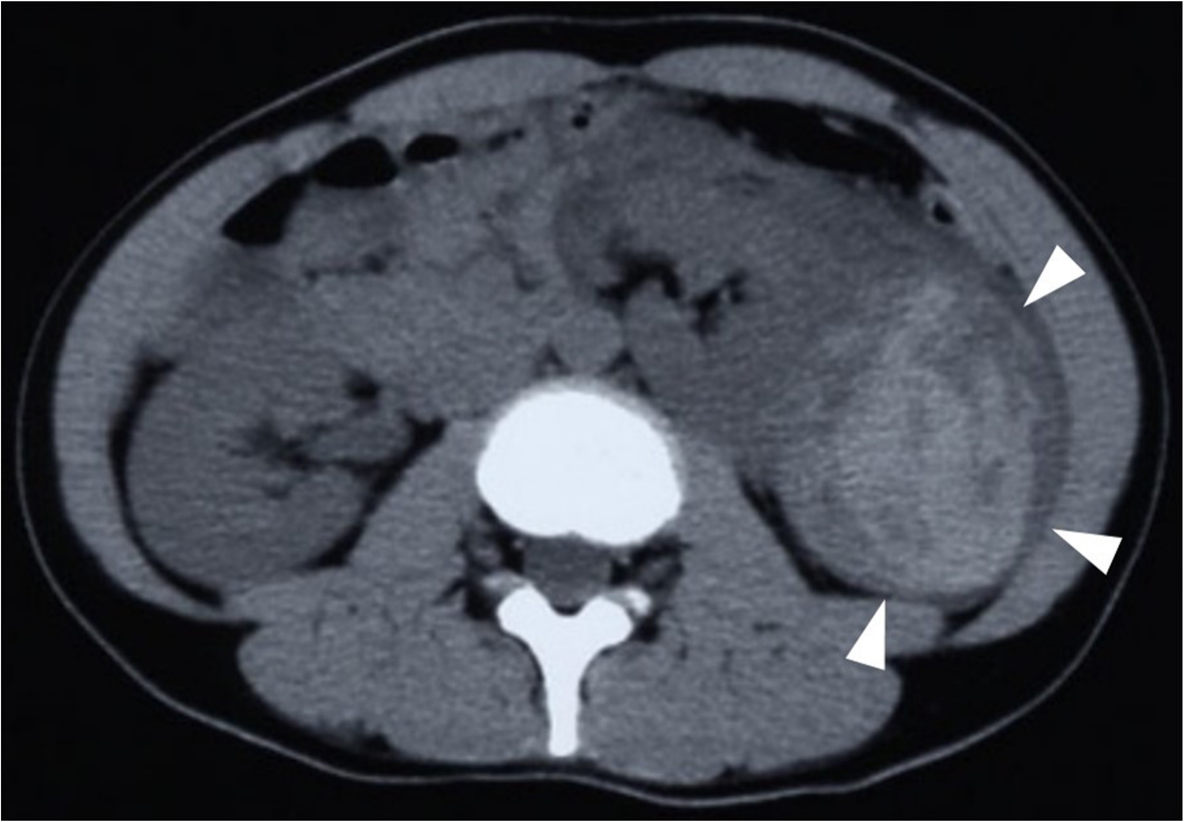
Enhanced abdominal computed tomography. An abdominal computed tomography image showing a hemorrhagic cyst in the upper pole of the left kidney (arrowheads).
